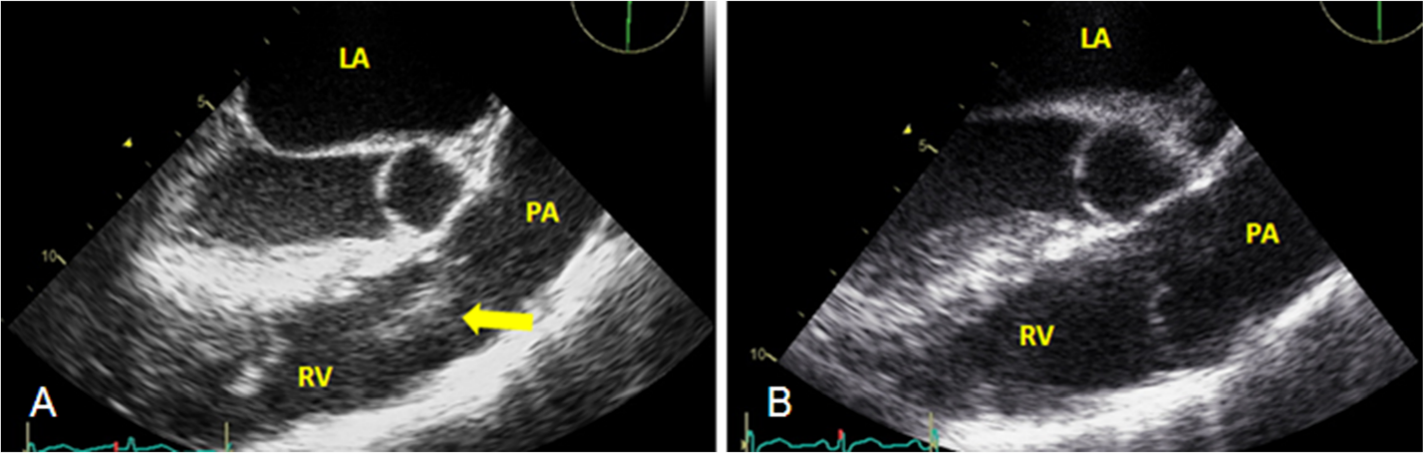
Transesophageal echocardiogram. A. Pulmonary valve vegetation B. No vegetation after four weeks of Amphotericin B.
